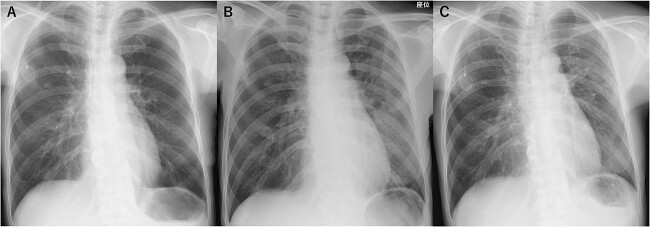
A series of chest radiographs. (A) On admission—no abnormalities other than a known lung metastasis in the right middle lung region. (B) Day 20—infiltrative shadow in the left middle lung region. (C) Day 34—shrinkage of infiltrative shadow.
